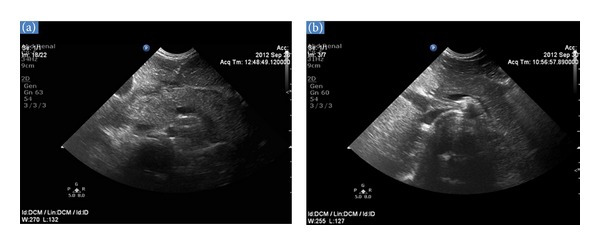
(a) Abdominal ultrasound on admission. Upper abdominal transverse section showing generalized enlargement of the pancreatic gland with a slightly heterogenous parenchyma and an overall reduction in reflectivity. Peripancreatic fluid collections are also noted. (b) Abdominal ultrasound on the 5th hospital day. Upper abdominal transverse section showing normalization of the size of the pancreas and complete resorption of the peripancreatic fluid collections.
